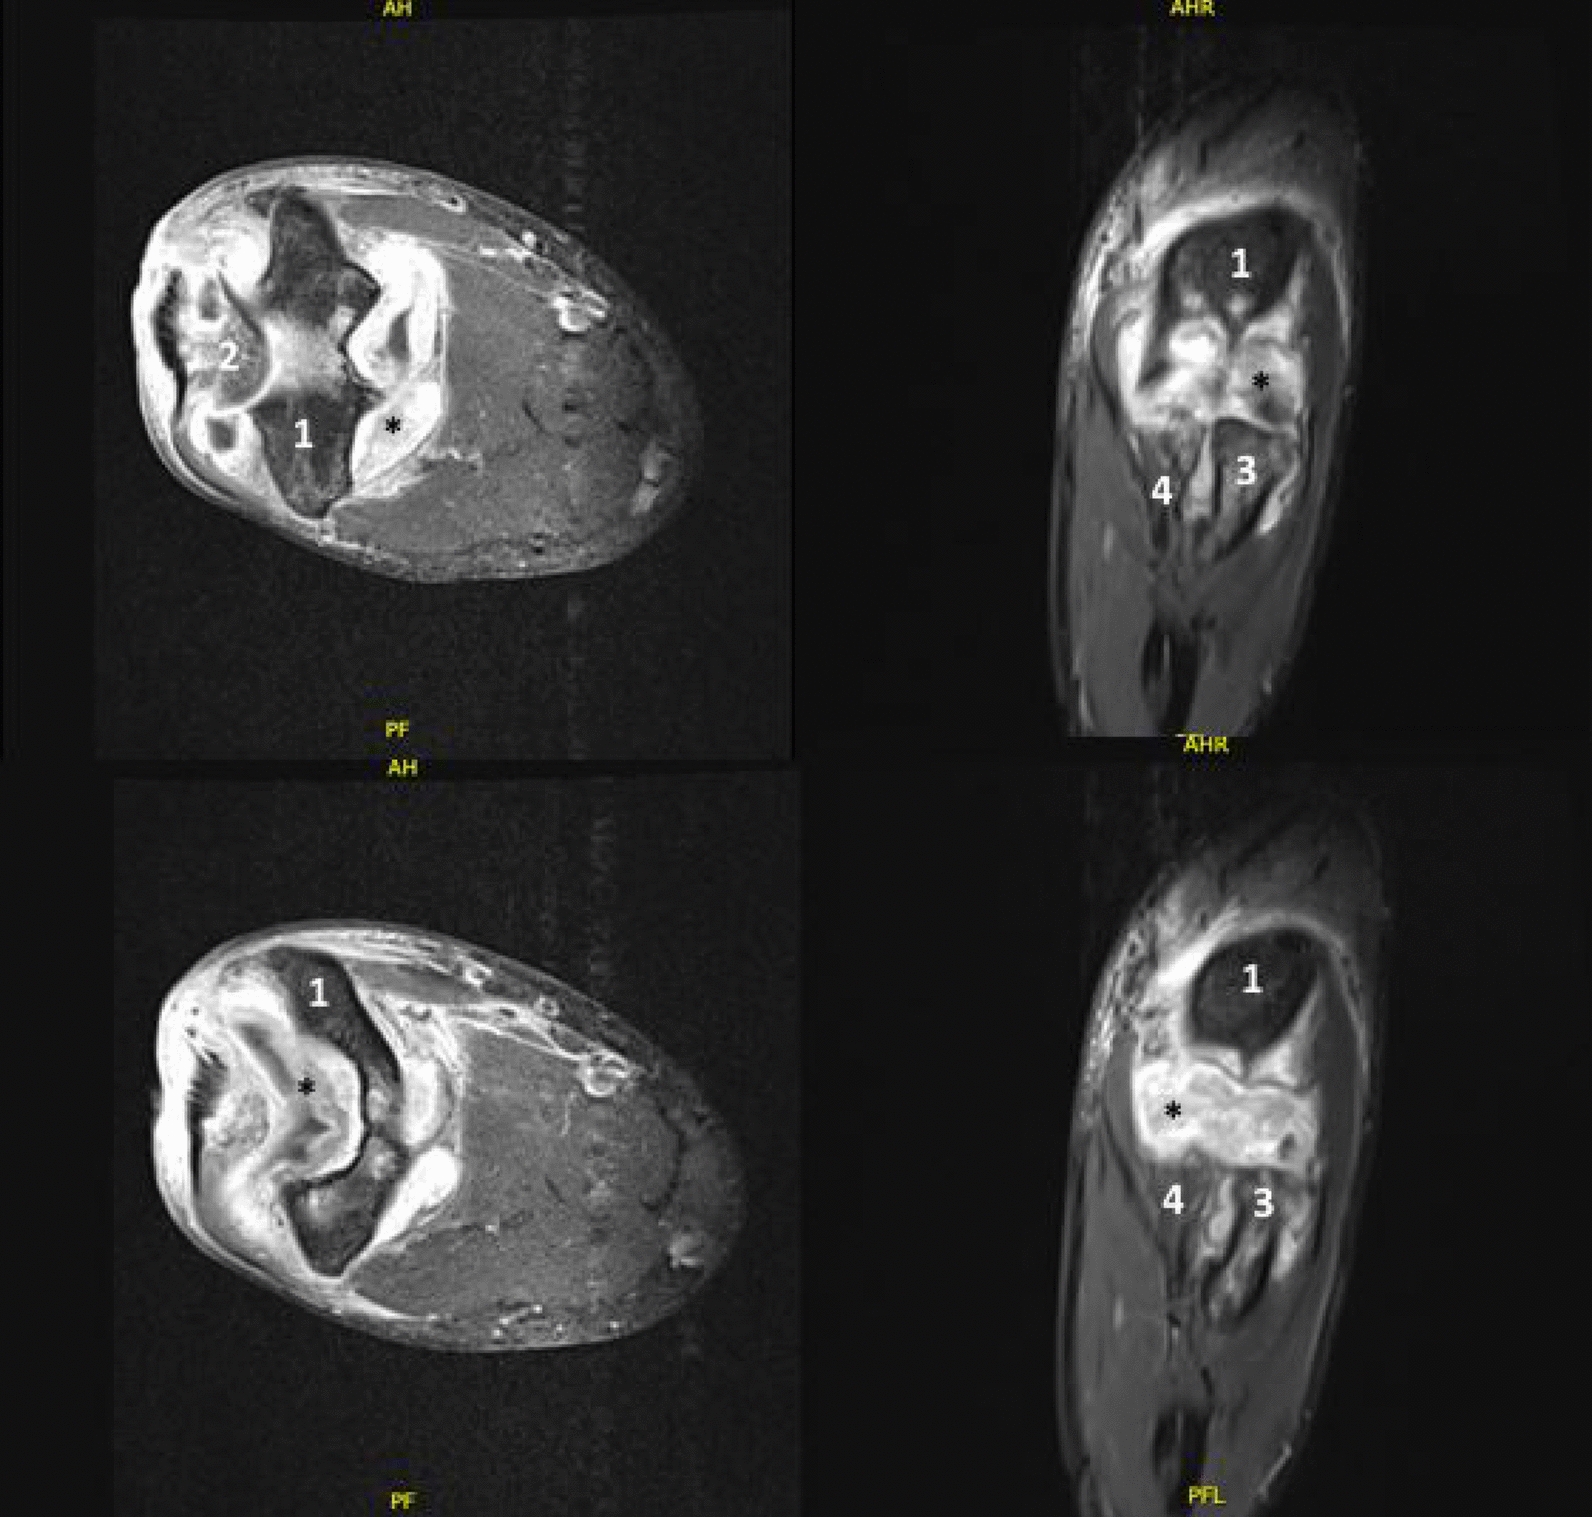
Magnetic resonance imaging scan of the elbow 21 weeks after initial clinical examination: 1, humerus; 2, olecranon; 3, radius; 4, ulnae; *shows synovial inflammation
